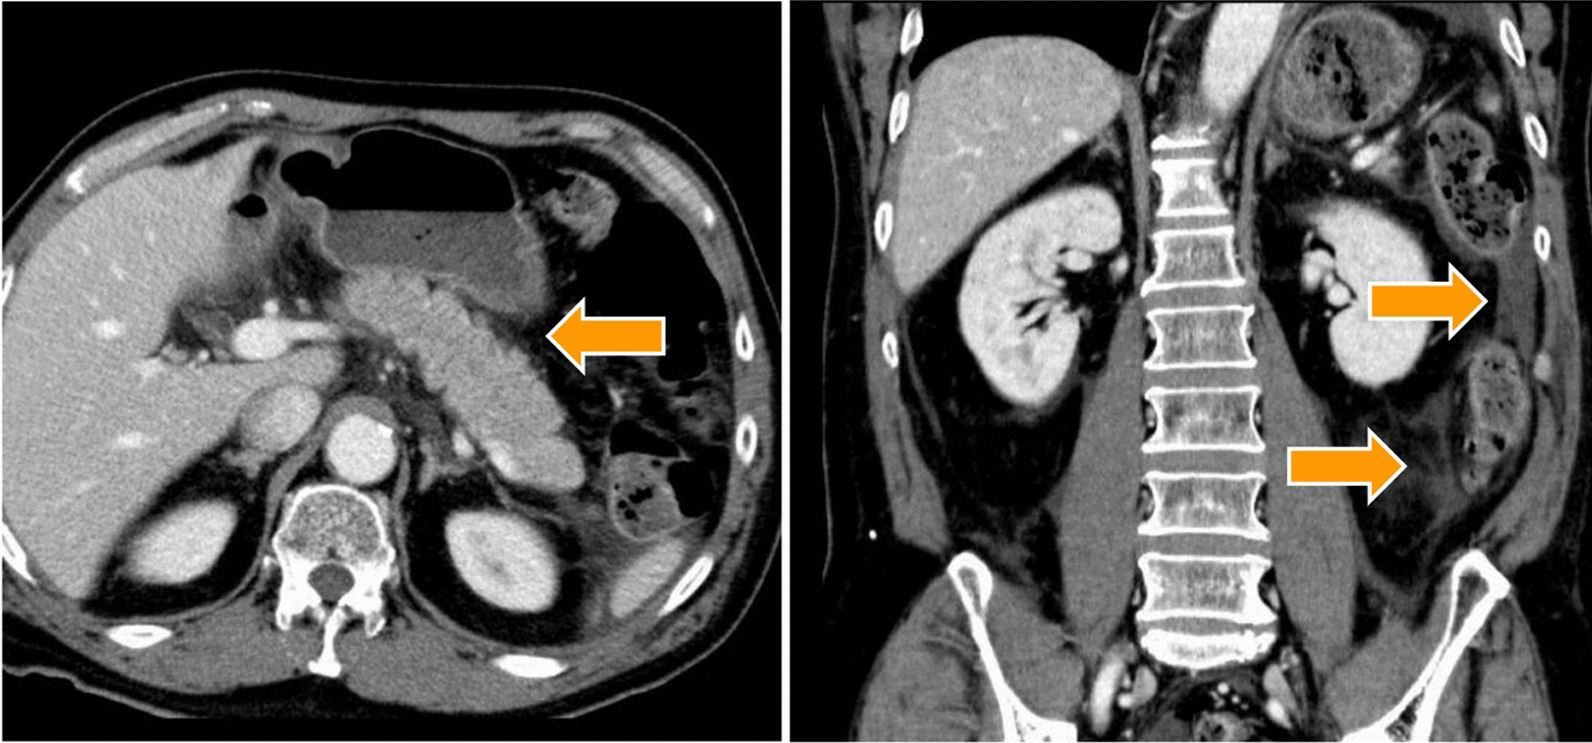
Contrast-enhanced abdominal computed tomography scan. Contrast-enhanced abdominal computed tomography scan shows diffuse pancreatic enlargement (arrow on axial plane) and inflammation extending beyond the sub-renal pole and beyond (arrow on coronal plane). There is no contrast failure zone, suggesting contrast computed tomography grade 2 acute pancreatitis
